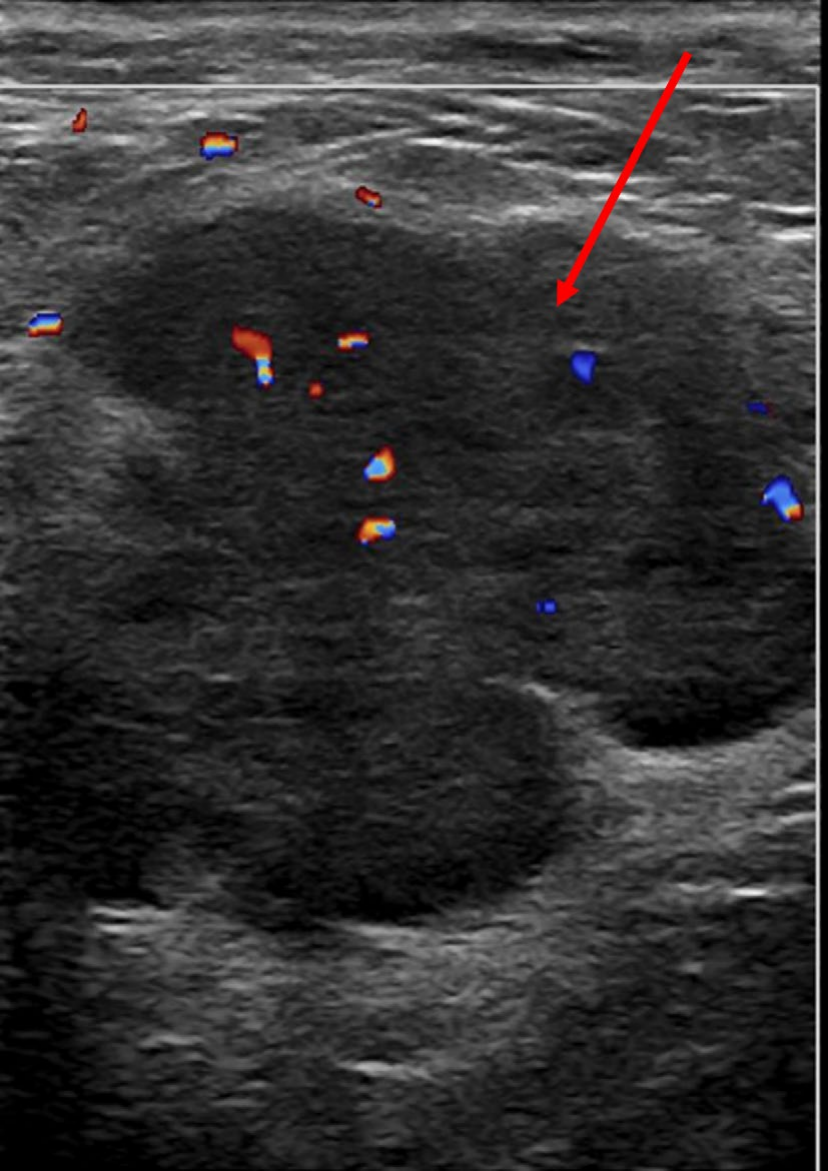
Transabdominal ultrasound of the suprapubic region performed the same day as the CECT. The image demonstrates a hypoechoic irregular mass with lobulated borders, internal vascularity on color doppler (red arrow), and the lesion measured 4.7 × 4.2 × 3.2 cm.
